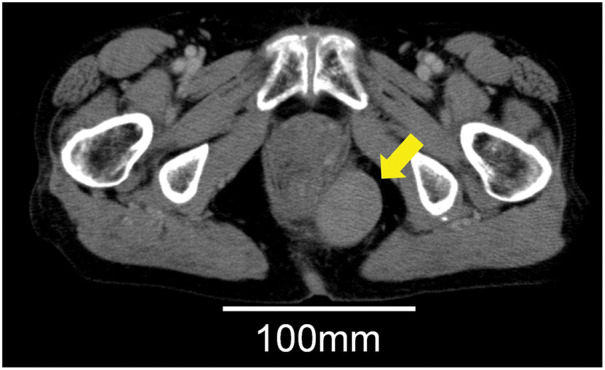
Contrast-enhanced CT (CECT) revealed a 46 × 28 mm mass on the left dorsolateral aspect of the lower rectum (yellow arrow).
